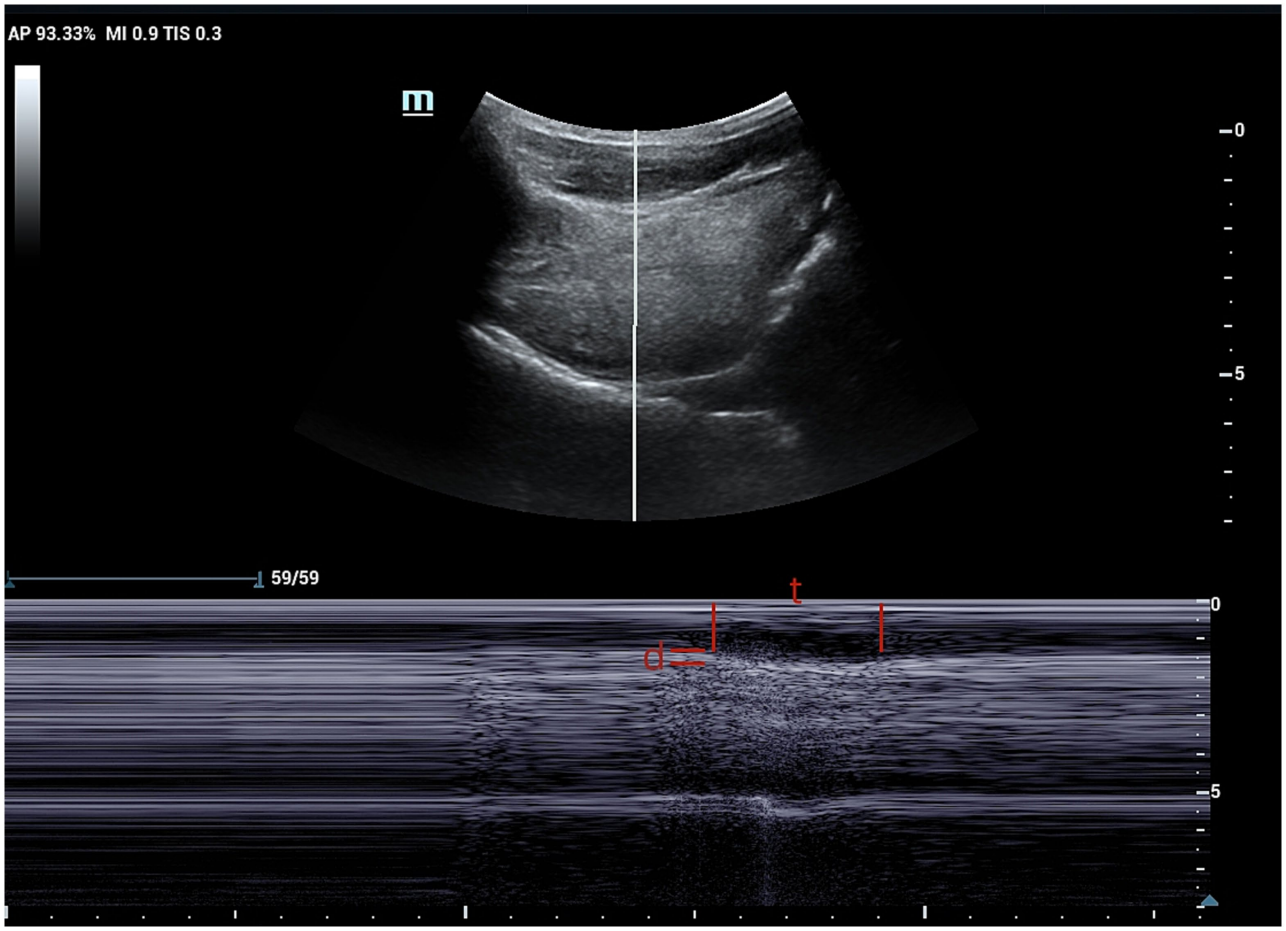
M-mode ultrasound image showing time and distance of genioglossus movement. The sampling line is placed at 2 cm from the hyoid bone. t, time of genioglossus movement; d, distance of genioglossus movement.
